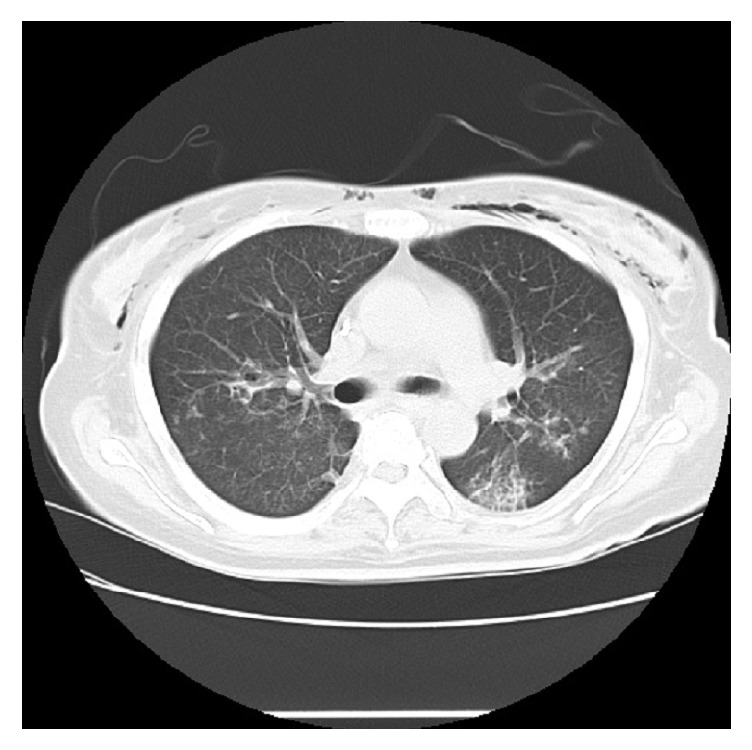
CT scan after extubation. The pulmonary infiltrates and ground-glass opacities had disappeared.
